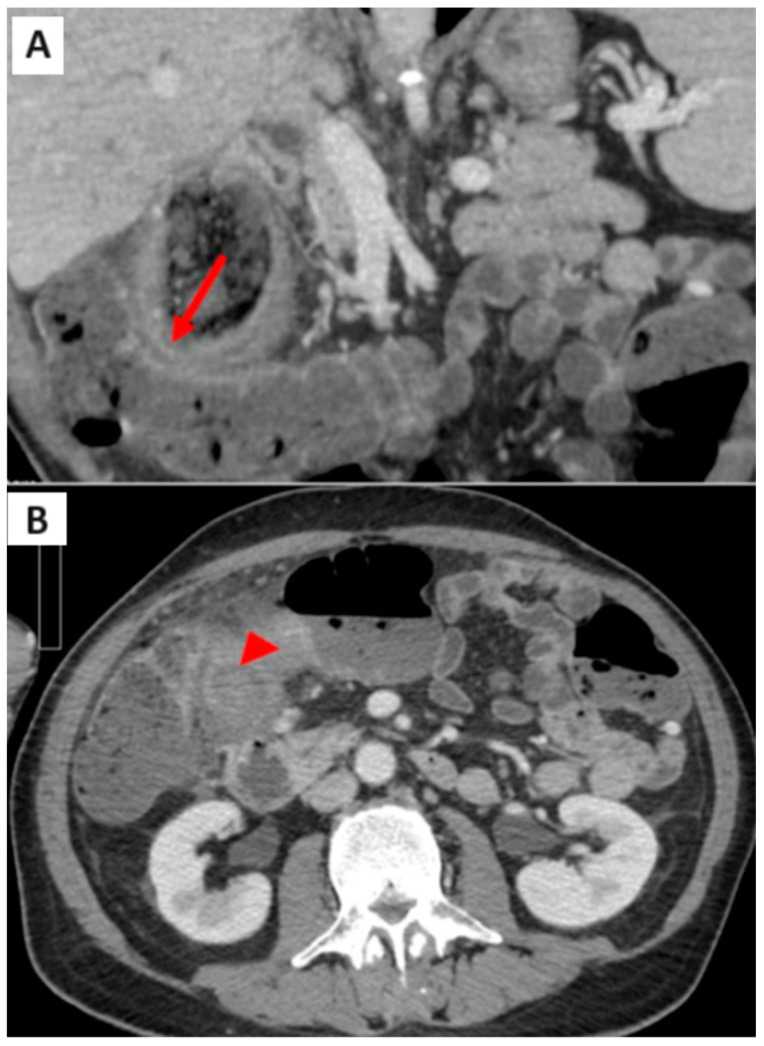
(A) CT scan: proximity of gallbladder wall, pericholecystic collection, and right colonic flexure (arrow); (B) CT scan: fat stranding of the pericholecystic tissue (arrowhead).
